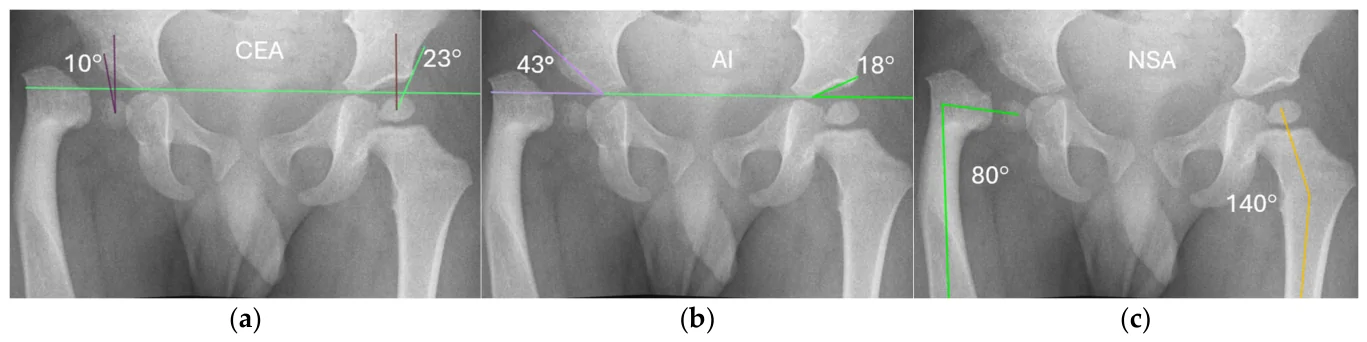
Radiographic hip measurements: (a) center-edge angle (CEA), normal ≥ 20°; (b) acetabular index angle (AI), normal ≤ 30°; (c) neck-shaft angle (NSA), normal ~ 130°.
